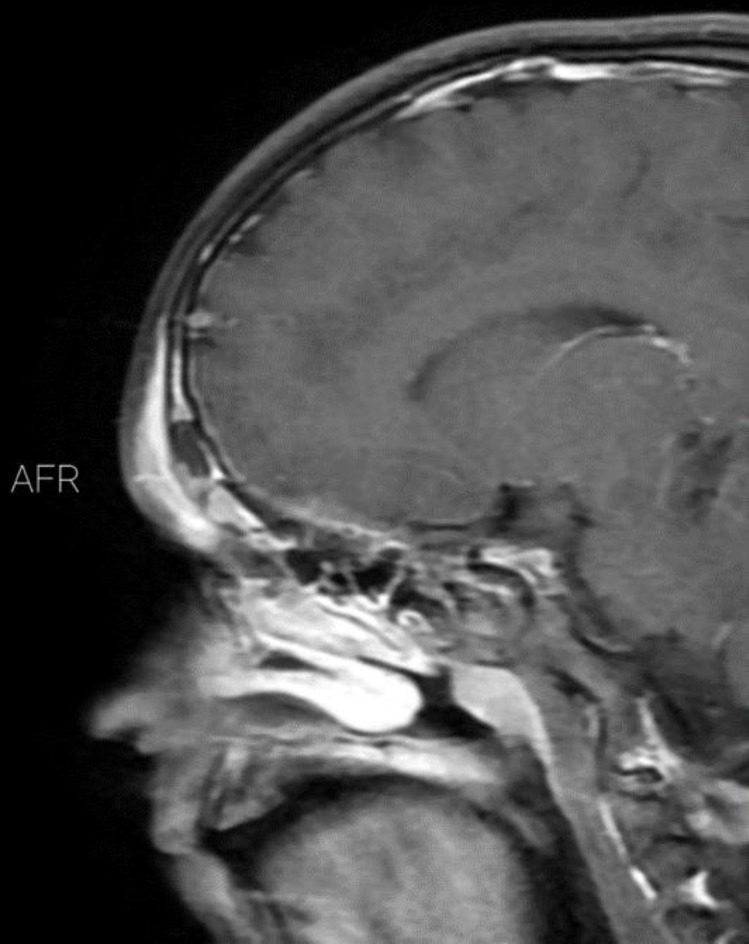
Sagittal T1 magnetic resonance imaging of the head showed mass in frontal sinus and inflammation in soft tissue of forehead
